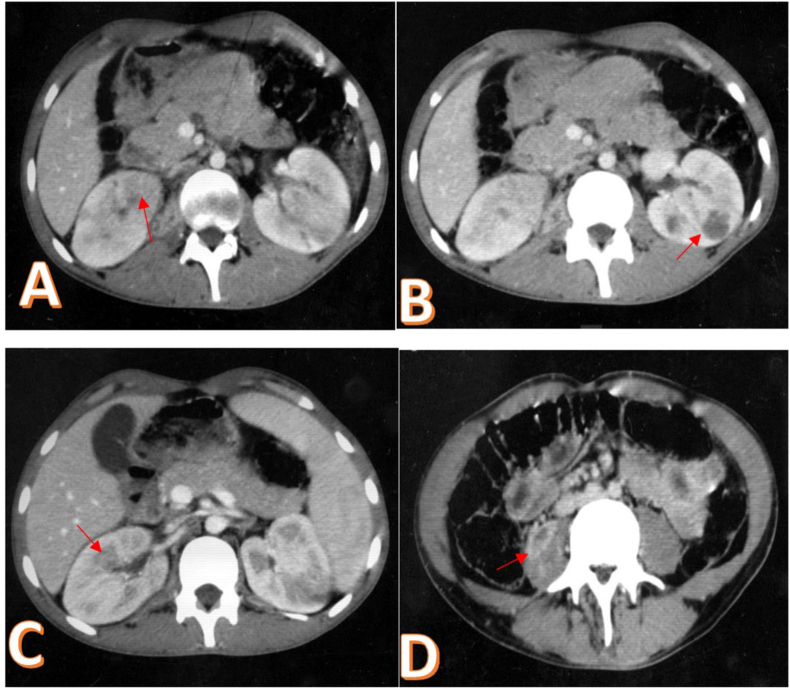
Control CT scan: Clear regression of collections on both sides.
